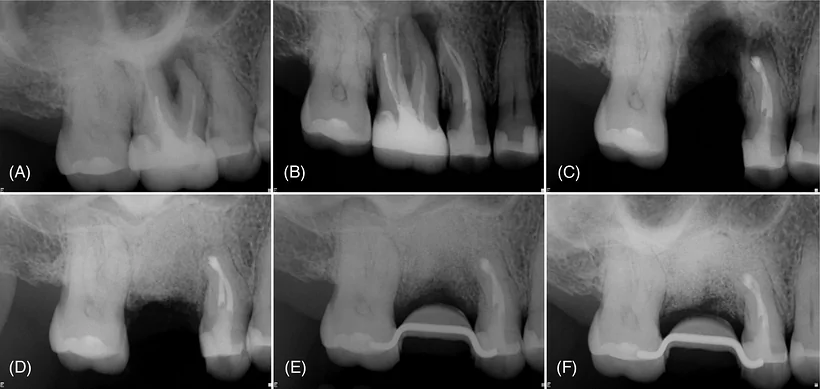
Case 2: Periapical radiograph showing a radiolucent lesion involving teeth #3 and #4 (A). Periapical radiograph after root canal treatment of tooth #4 (B). Intraoperative radiograph following the extraction of tooth #3 and retrofilling of tooth #4 (C). Immediate postoperative radiograph after guided bone regeneration (D). Radiograph at the 1‐month follow‐up, taken after provisional restoration placement for tooth #2 (E). Radiograph at the 8‐month follow‐up (F).
